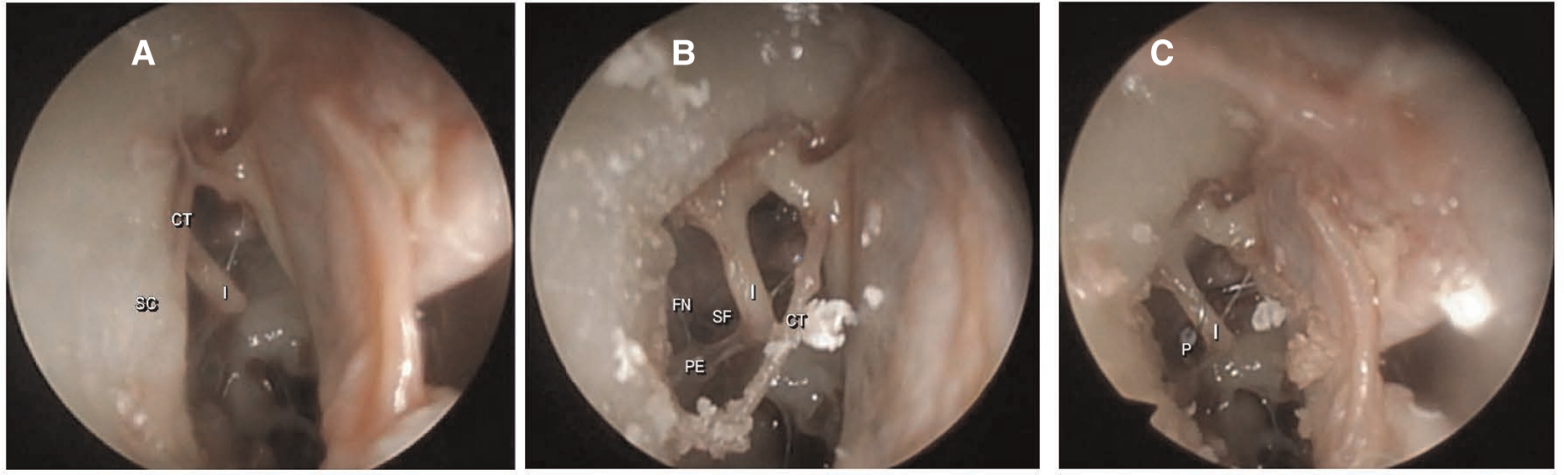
Endoscopic photographic view of the middle ear landmarks using a 4 mm endoscope with a 30° angle. (A) After dissection of the tympanomeatal flap; CT (chorda tympani), I (long process of the incus) and SC (scutum). (B) After scutum removal; PE (pyramidal eminence), FN (facial nerve) and SF (stapes footplate). (C) After prosthesis placement; *P* (prosthesis).
